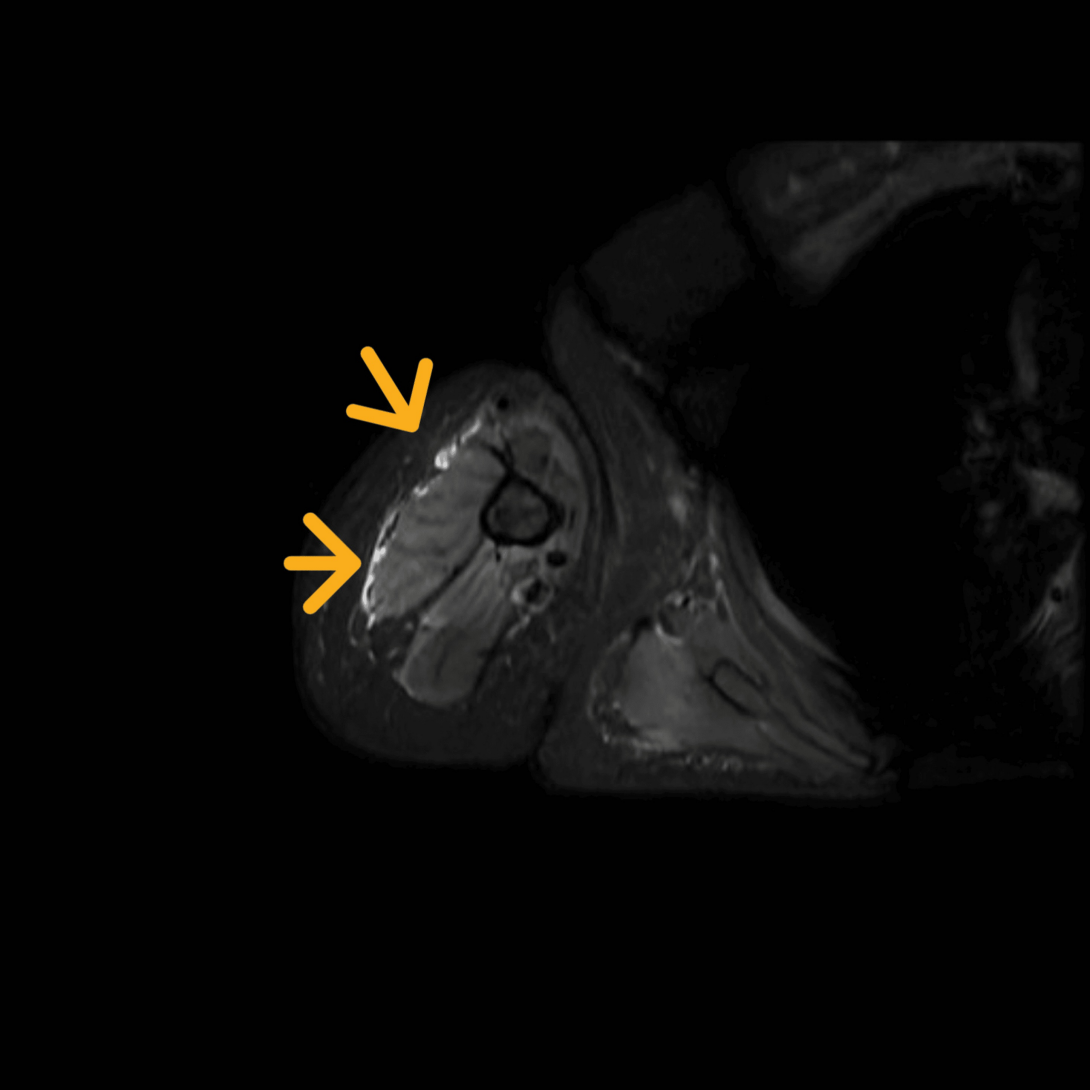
MRI of the muscles of the arm STIR sequence showing hyperintensity in the arm muscles (yellow arrows). STIR, short tau inversion recovery
